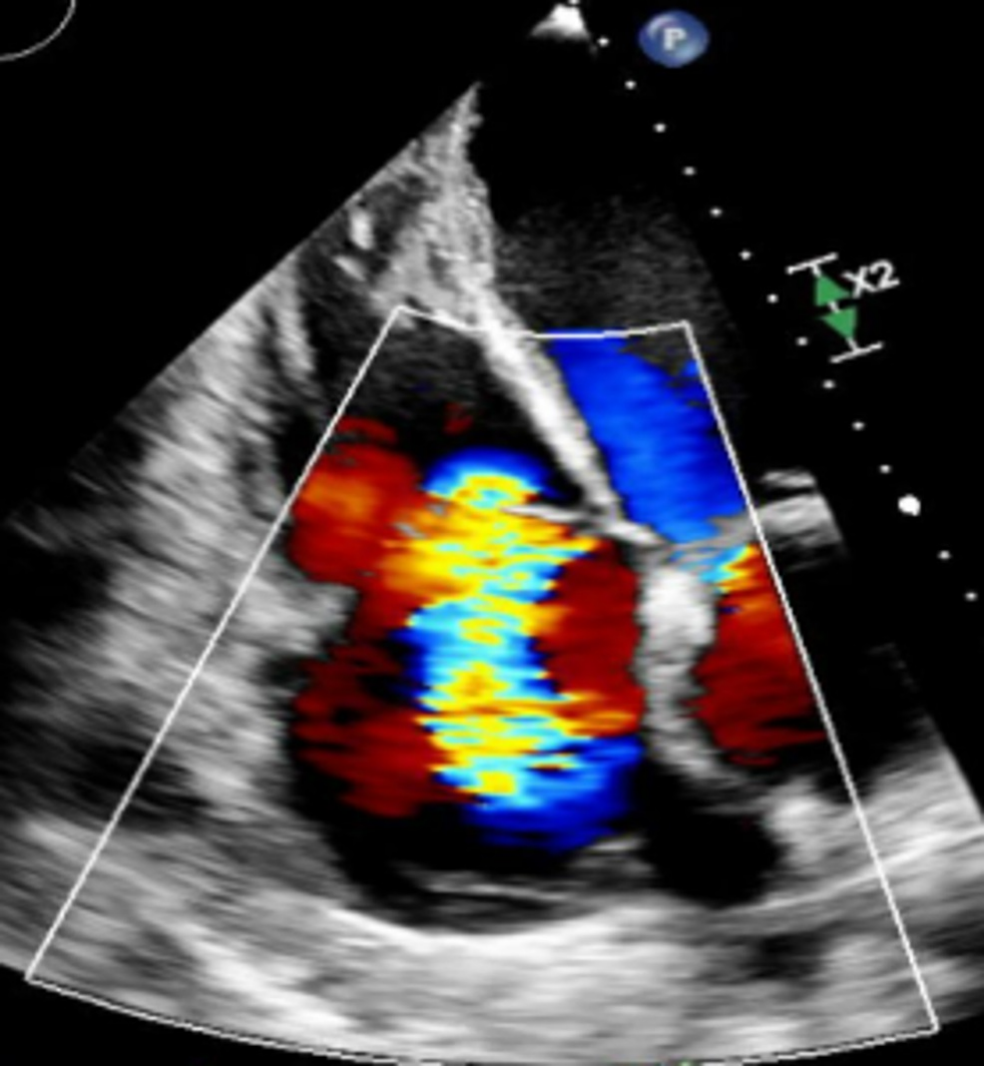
Tricuspid Regurgitation (four-chamber view on echocardiogram)
